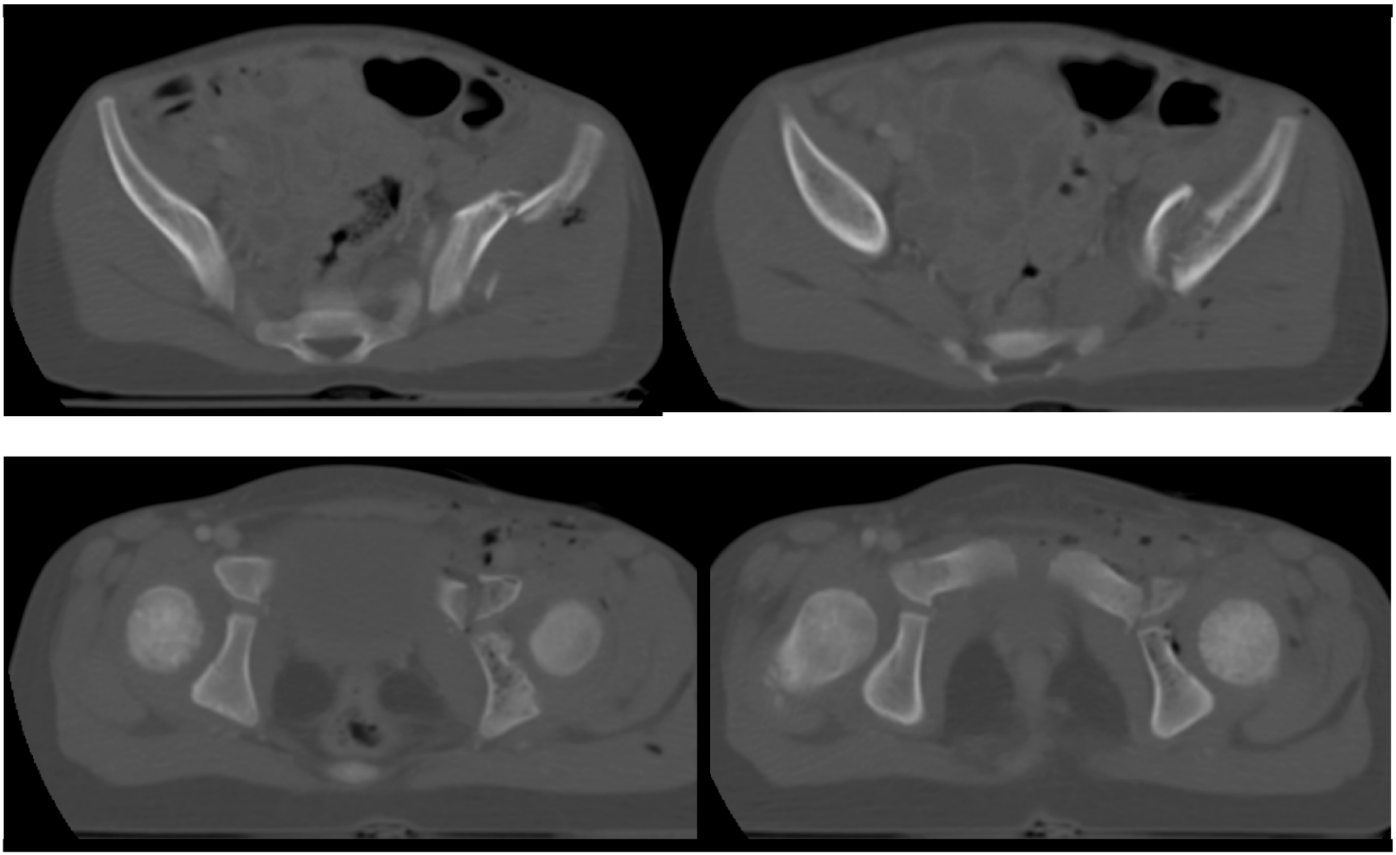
Axial CT scan at day of injury revealing a left anterior column acetabular fracture which involves the tri-radiate cartilage.
